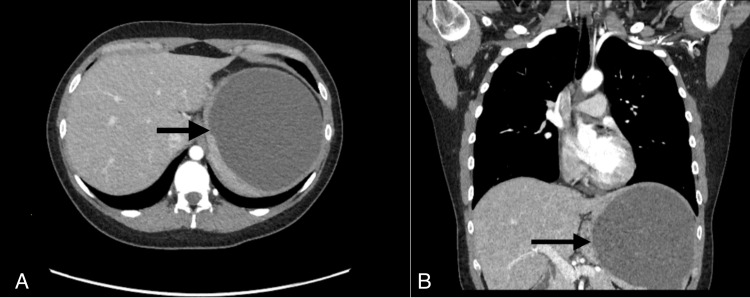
Axial (A) and coronal (B) views of abdominal CT scan showing a large hyper-dense area of a hydatid cyst (black arrows).
